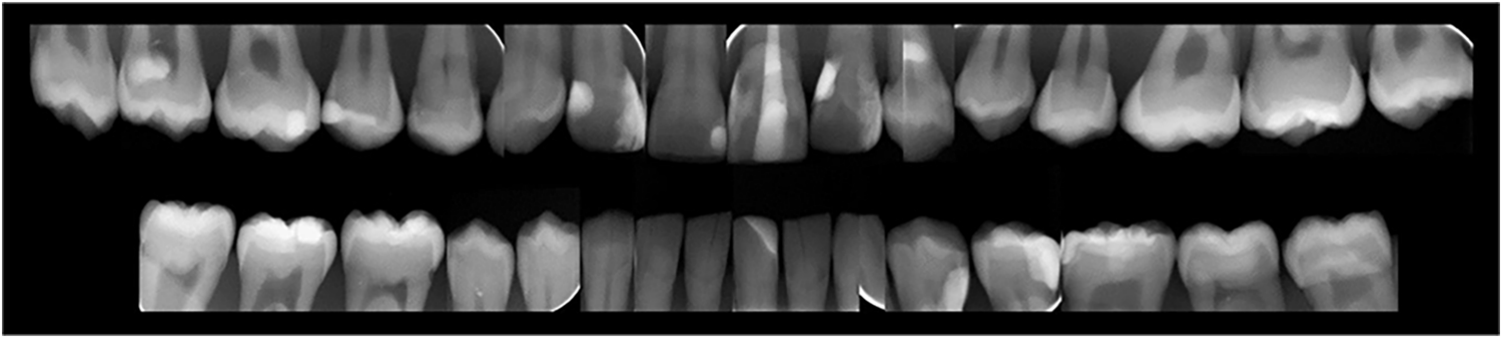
Intraoral radiography status of the crowns of the tooth model
